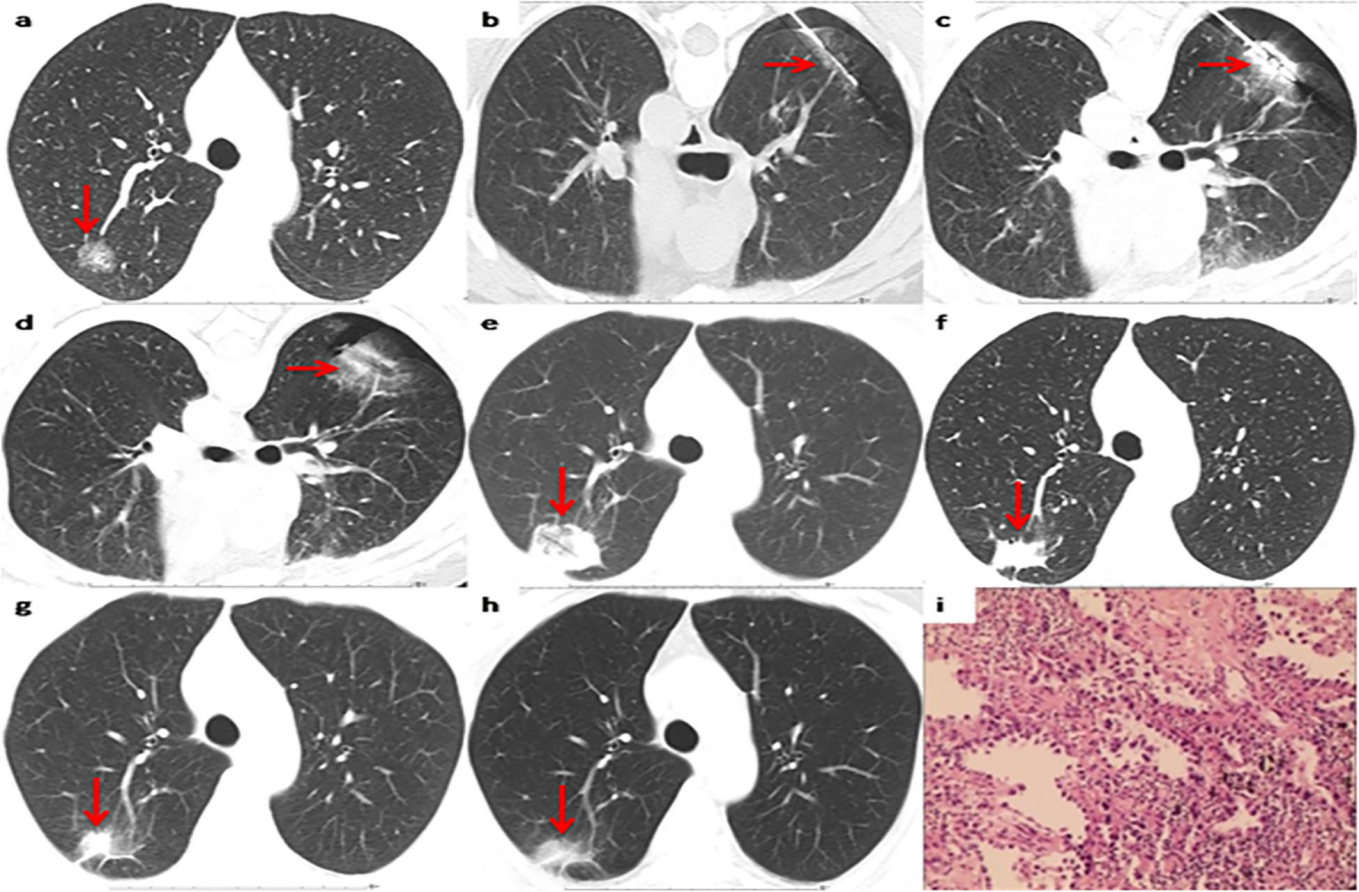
Representative CT scans of a patient with primary lung invasive adenocarcinoma after breast cancer surgery. (a) Preoperative image. (b) The biopsy needle has been inserted into the nodule center to complete the biopsy sectioning. (c) The MWA antenna has been inserted into the nodule center. (d) Postoperative image immediately after MWA. (e) 1 month after MWA. (f) 12months after MWA. (g) 36 months after MWA. (h) 60 months after MWA. (i) Pathological results of primary lung invasive adenocarcinoma biopsy.
